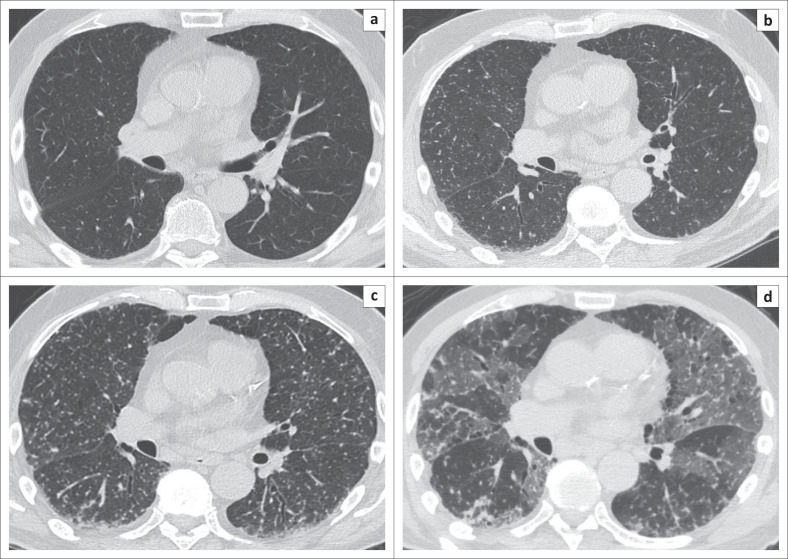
A normal CT of the chest performed before the patient’s access to the Emergency Department (a). A CT scan of the same patient performed 10 days after the last Bacillus Calmette-Guérin instillation, showing 1 mm – 2 mm diameter nodules disseminated throughout the lung and distributed randomly with respect to the lobular structures in a miliary pattern (b). After two weeks of antibiotic therapy, CT imaging revealed a numerical and dimensional increase in the multiple micronodules (c). Despite the administration of anti-tubercular therapy, the patient’s clinical condition deteriorated again, requiring a new CT scan that revealed the presence of bilateral ground-glass opacities localised mainly in the middle and lower parts of the lungs, partially superimposed on the previously seen multiple lung micronodules, compatible with coronavirus disease 2019 superinfection (d).
